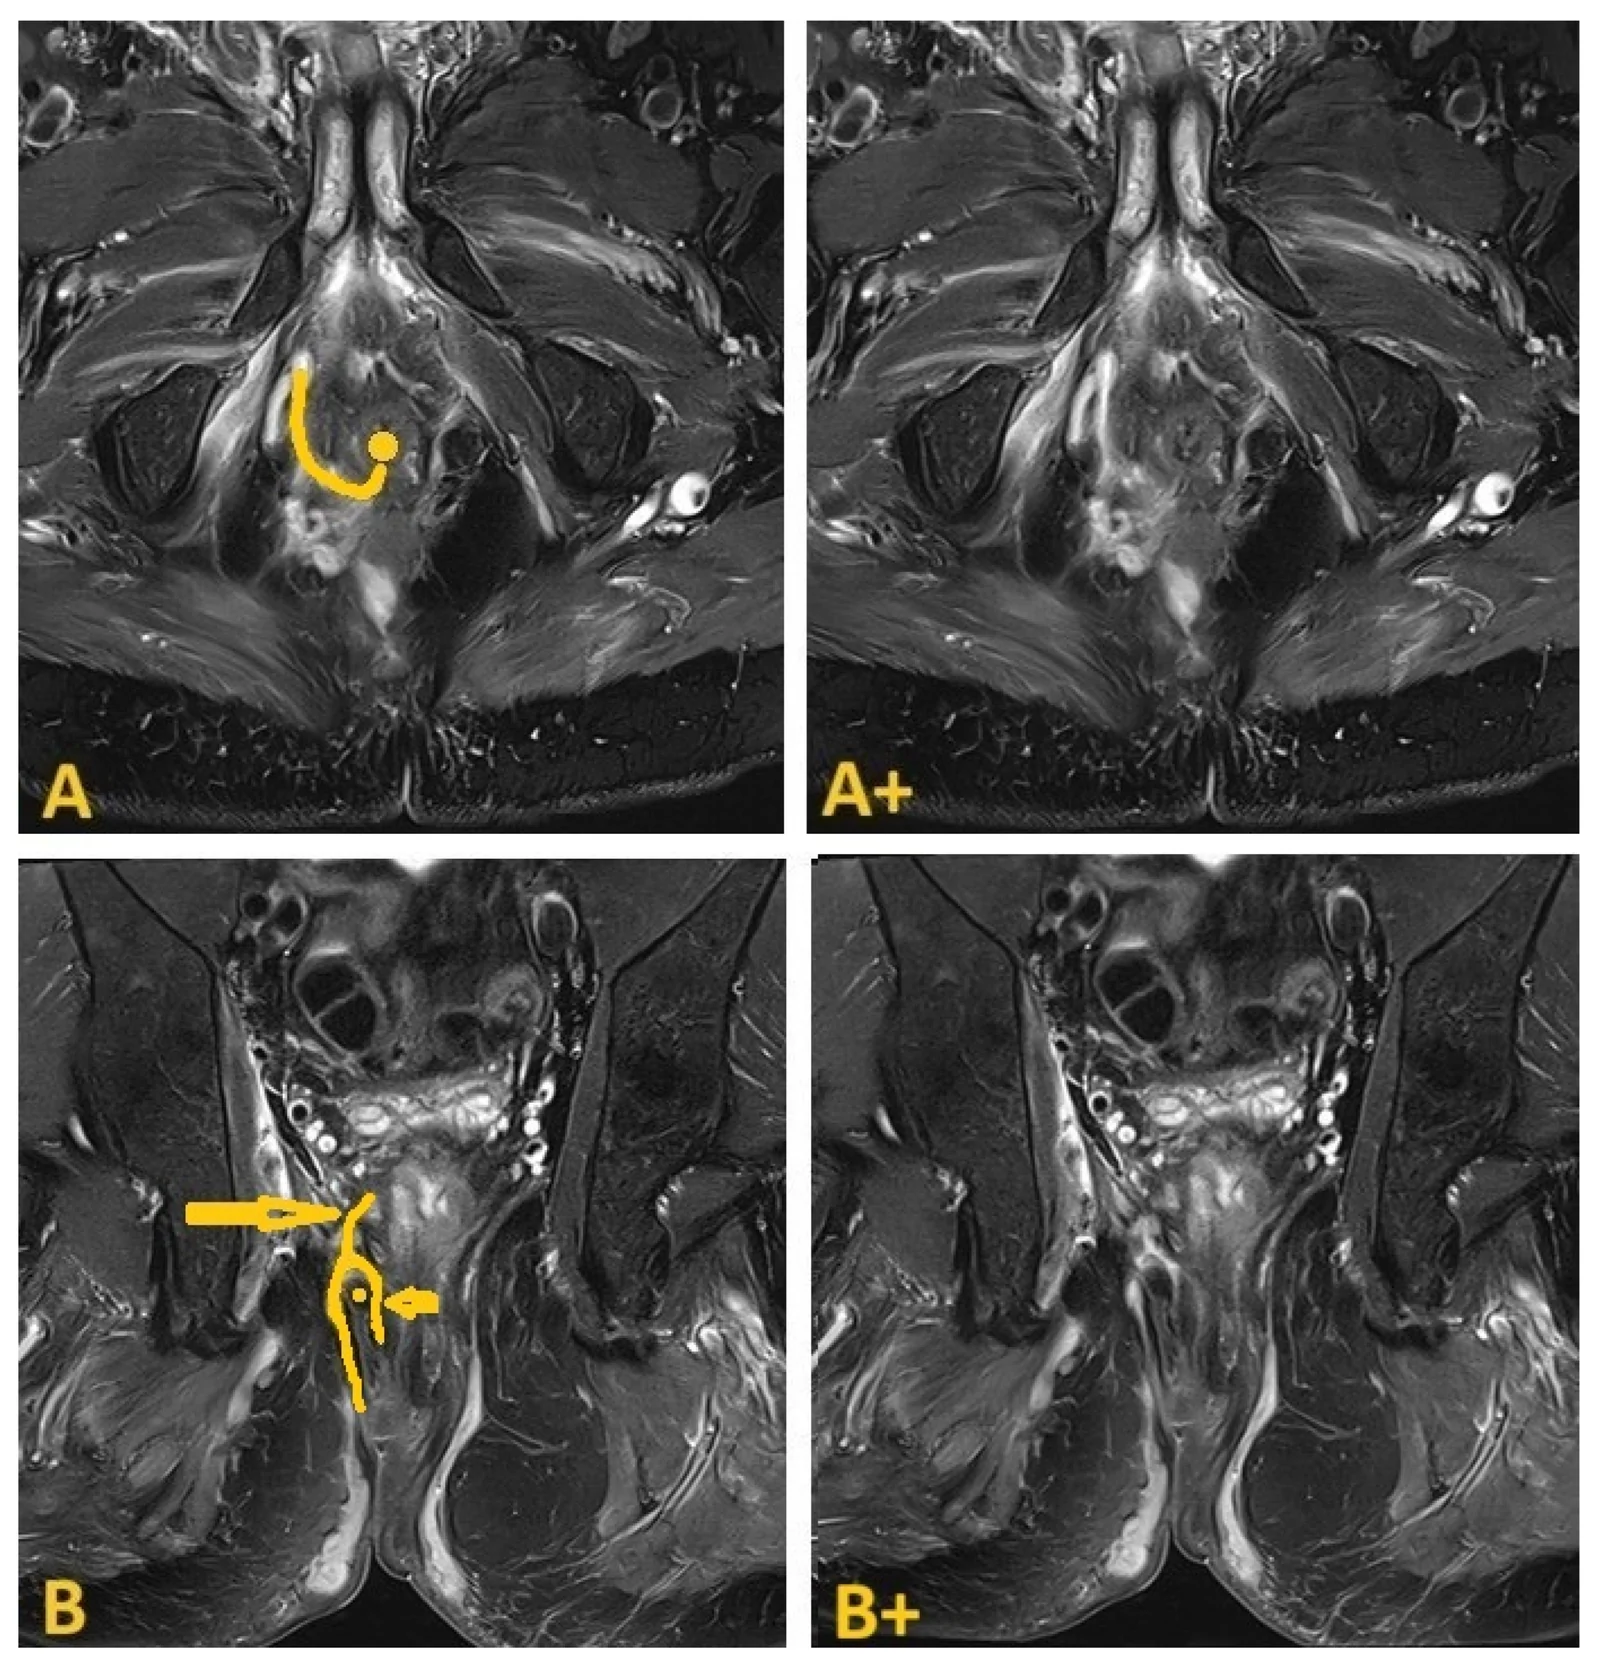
Selected magnetic resonance images. (A) Axial fat-suppressed T2-weighted image showing the internal opening of a perianal fistula arising from the posterior midline anal sphincter region at the 6 o’clock position. (A+) Corresponding unannotated image. (B) Coronal fat-suppressed T2-weighted image showing a solitary fistulous tract extending superior to the puborectalis muscle (dot) before dividing into two branches. One branch forms a suprasphincteric tract (short arrow) coursing inferiorly towards the anal canal and opening at the 6 o’clock position, while the second branch extends superiorly through the levator ani muscle to form an extrasphincteric tract (long arrow) opening into the rectum. (B+) Corresponding unannotated image.
